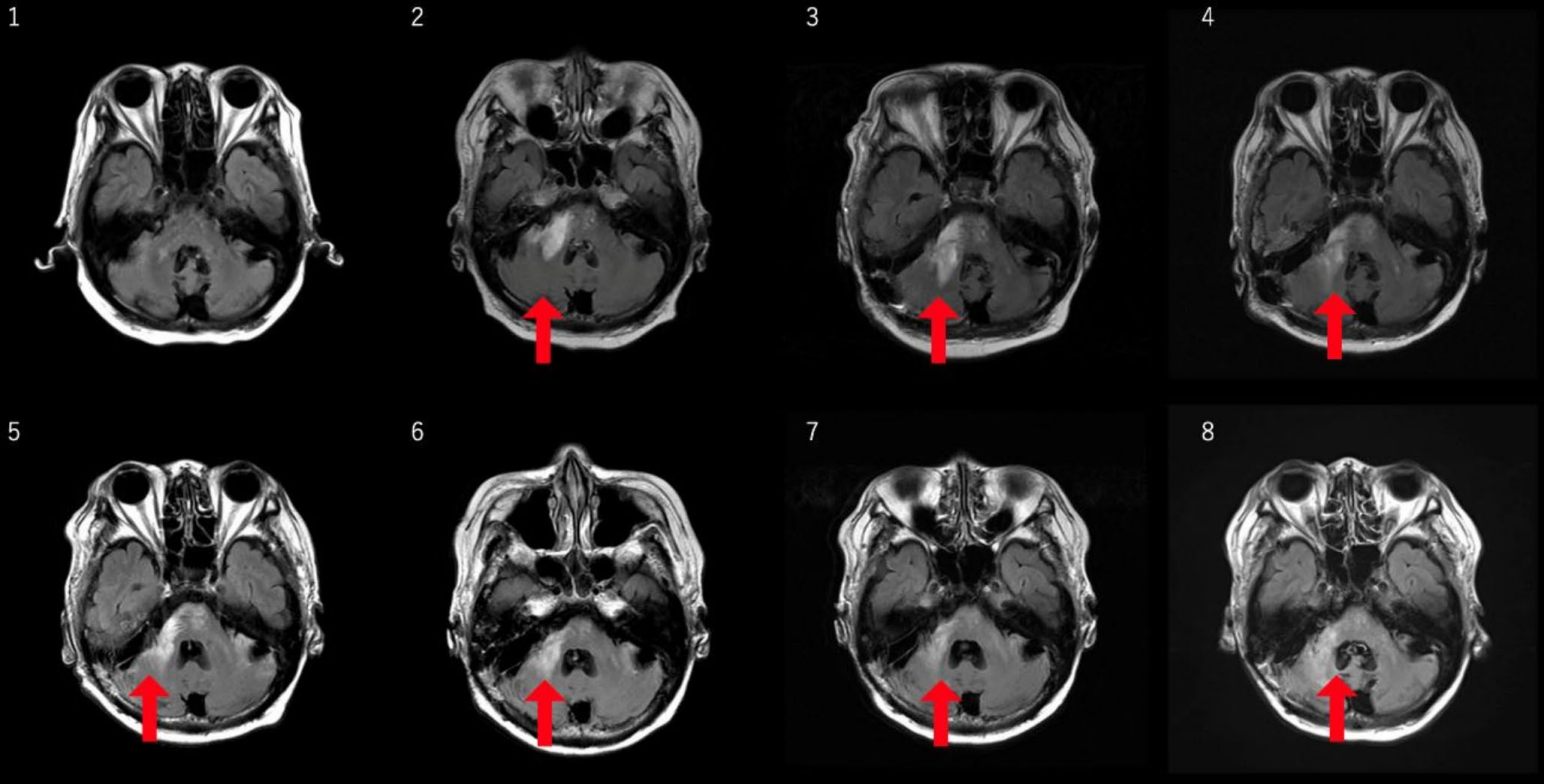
Axial fluid-attenuated inversion recovery (FLAIR) images with contrast enhancement
